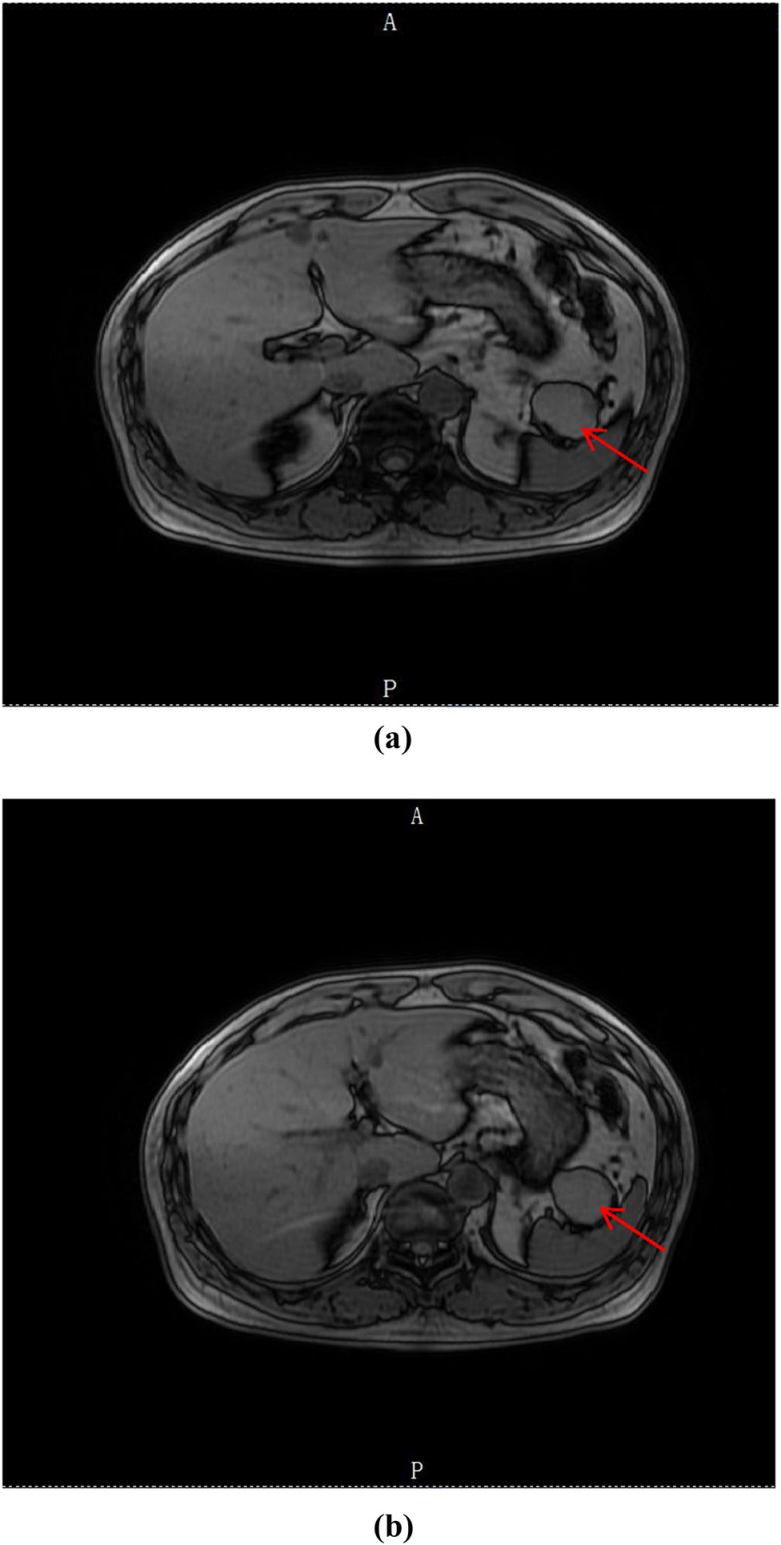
(a, b) MRI scan of the abdominal space occupying lesion. The red arrow shows nearly-circular mass with equal T1 and short T2 signal.
